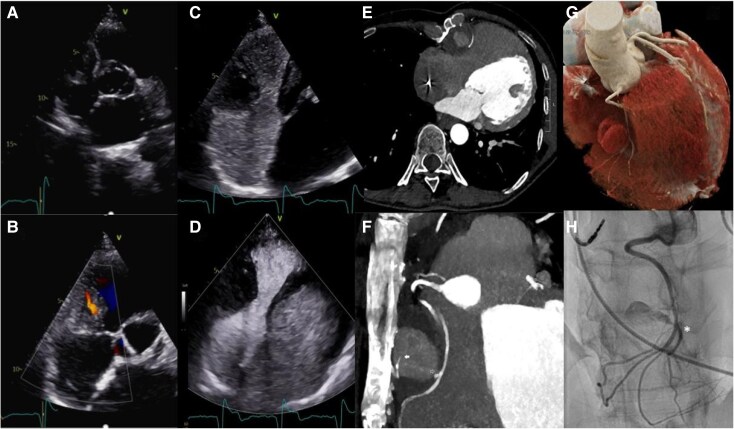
Multimodality approach to mediastinal mass: functional and anatomical consideration. (*A*) Parasternal short-axis view displaying a mediastinal mass adjacent to the right atrioventricular groove. (*B*) Low-velocity colour Doppler application demonstrating systolic–diastolic flow within the lesion, with suspected circumferential thrombosis. (*C*) Agitated saline contrast echocardiography filling the right heart chambers and excluding communication between the right-side heart and the mass. (*D*) Contrast-enhanced ultrasound demonstrating progressive opacification of the cardiac chambers (before right side, then left side), followed by delayed enhancement of the mass, suggesting an arterial supply from the systemic circulation. (*E*) Transversal-axis CT excluding other mediastinal aetiologies, such as pericardial or bronchogenic cysts, displaying the markedly malaligned sternum, the concomitant pericardial thickening, and the secondary close contact between the mass and sternal wire. (*F*) Multiplanar reconstruction (MPR) vertical long-axis view excluding anomalous course of the RCA course (i.e. interatrial course) and confirming the anatomic relationships between the RCA, severely kinked by the mass in the mid-to-distal part, the blood supply, and the sternal wires (white arrows). (*G*) Three-dimensional (3D) volume rendering image clearly displaying the anatomical site of the suspected pseudoaneurysm and the close relationship with the RCA. (*H*) Invasive coronary angiography showing the active arterial blood supply of the mass by the RCA (white *).
